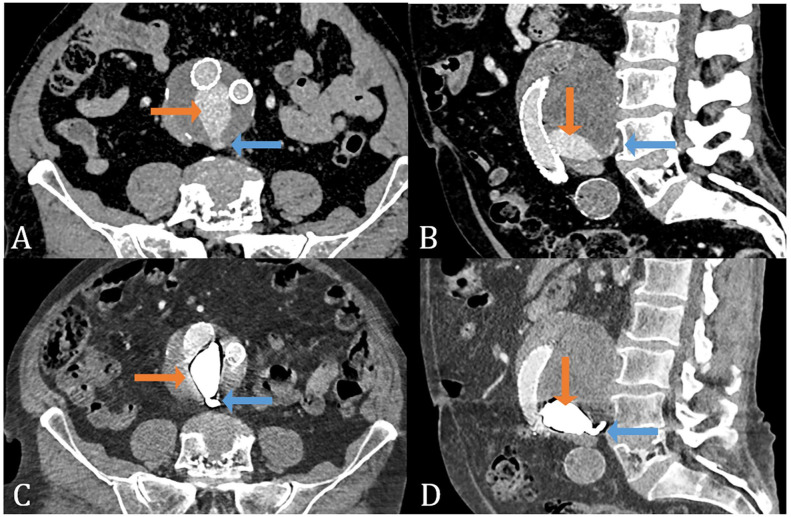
Preoperative computed tomography angiography (CTA) with type II endoleak (A,B) and connecting lumbar arteries (blue arrow) with endoleak cavity (orange arrow) and postoperative CTA with AneuFix treatment (C,D) and treated lumbar arteries (blue arrow) with treated endoleak cavity (orange arrow).
